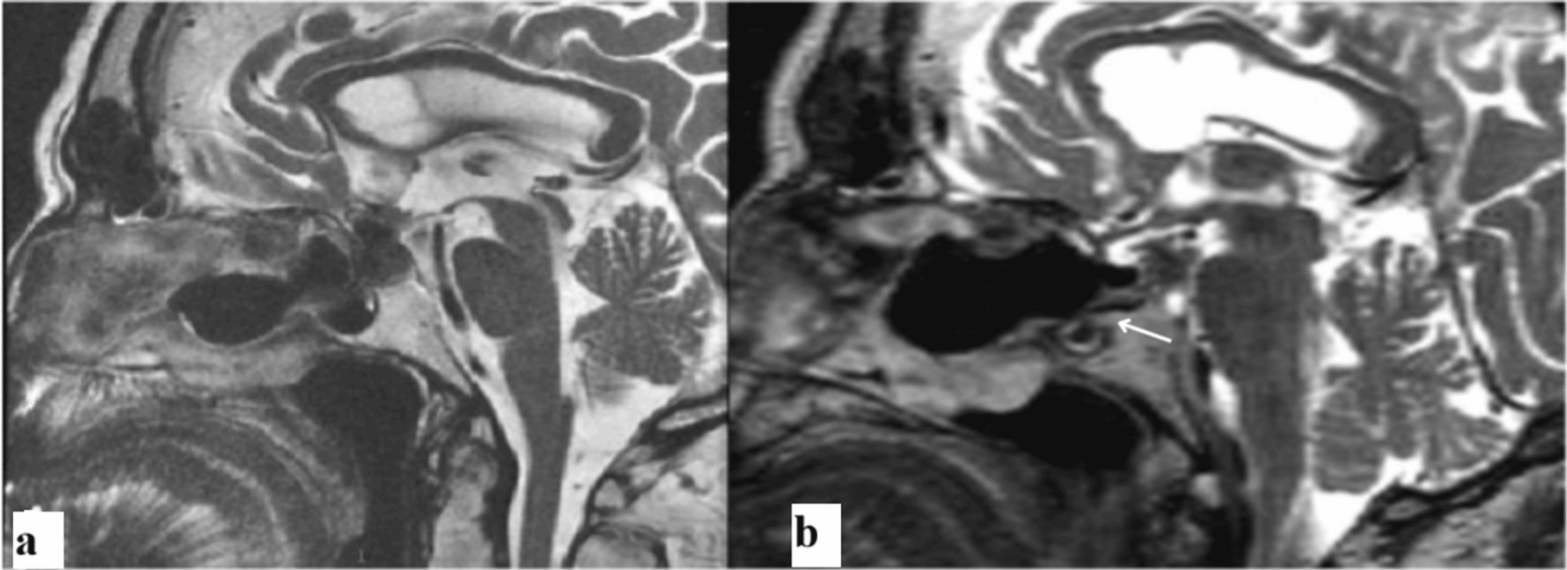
Magnetic resonance imaging T2-weighted sagittal sequences comparing pre- and postoperative findings (a) Presurgical image showing recurrent Rathke's cleft cyst with suprasellar extension and compression of the optic chiasm. (b) Eight-month postoperative image, demonstrating successful decompression of the optic chiasm with stable postoperative changes and no evidence of cyst reaccumulation. Communication between the Rathke's cleft cyst floor and the nasal cavity is shown using a white arrow
